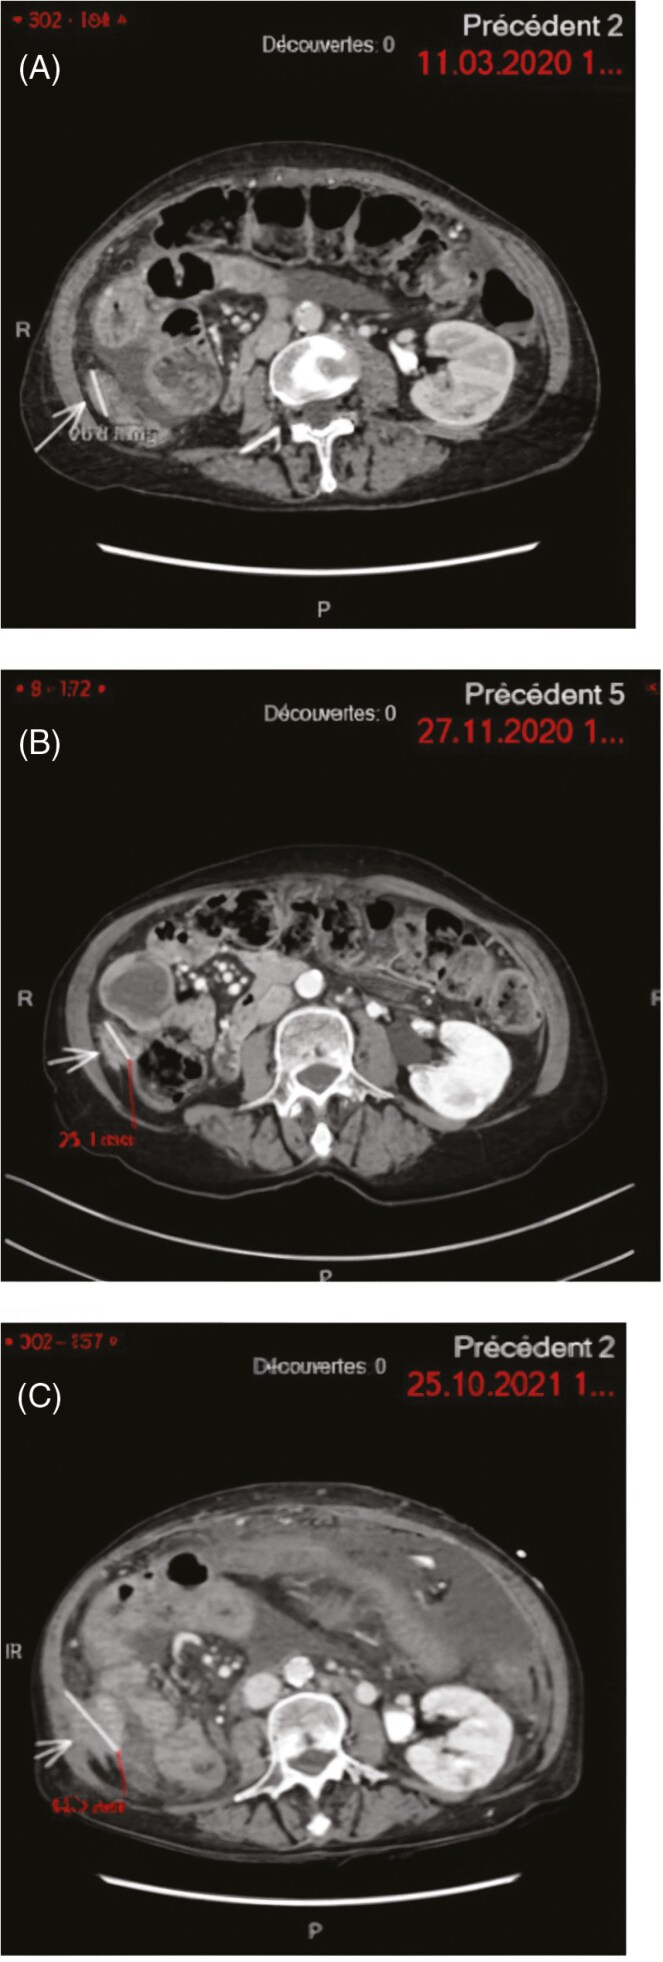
Peritoneal carcinosis target-lesion. MRI images showing the trajectory of the disease during specific time points. (A) March 11, 2020: Erdafitinib starting point, (B) November 27, 2020: Erdafitinib discontinuation due to toxicity—stable disease, (C) October 24, 2021: Pemigatinib starting point—progressive disease.
